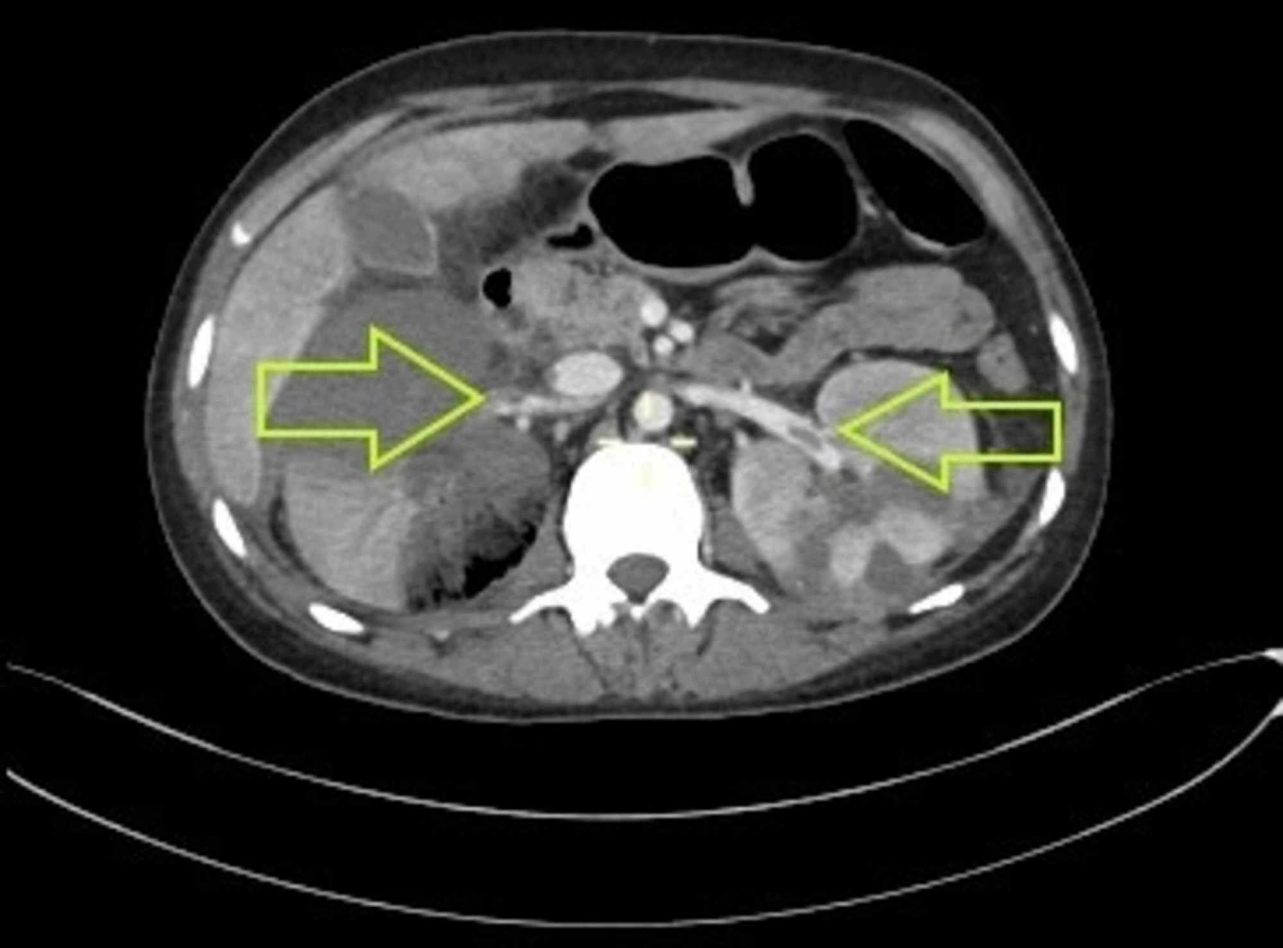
CT with contrast, transverse section showing bilateral renal vein thrombosis Wedge-shaped hypodensities seen in bilateral kidneys, more in the right kidney which is swollen and enlarged. Filling defect noted involving the right renal vein at its ostium and extending into the hilar region. At the same level, there is extension into the inferior vena cava as well. Similar filling defect is noted in the left renal vein at the hilum. Both filling defects are shown by the yellow arrows in the image.
